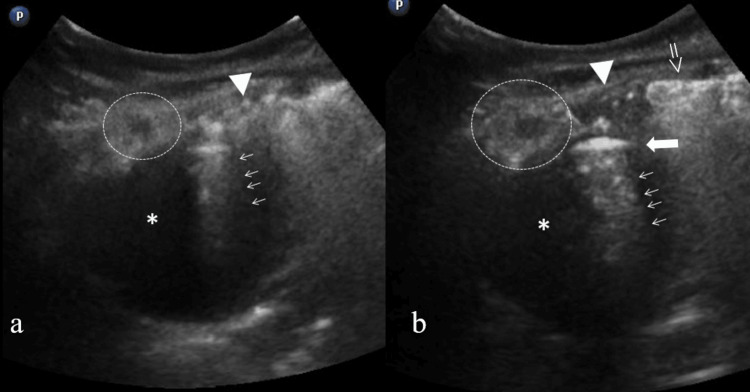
Lung ultrasound at the site of lesion Lung ultrasound (LUS) with prominent finding of an ovoid hypoechoic lesion (star: fluid-like appearance). Superiorly a dense hyperechoic arcuate-shaped area is present producing a comet-tail artifact (arrows) consistent with air having a linear inferior border (thick arrow in (b): air-fluid level). There is also a consolidated area (circle) with hypoechoic centre suspicious for necrotic pneumonia. Furthermore, superiorly to main lesion, in subpleural location, a hypoechoic area is present which looks like a jagged echoic edge and represents replaced by inflammatory exudate area, also known as “shred sign” (arrowhead). Finally, adjacent to “shred-sign” area is a hyperechoic subpleural region with extension throughout the image’s field, which represents “coalescent B-Lines” (open arrow in (b)).
